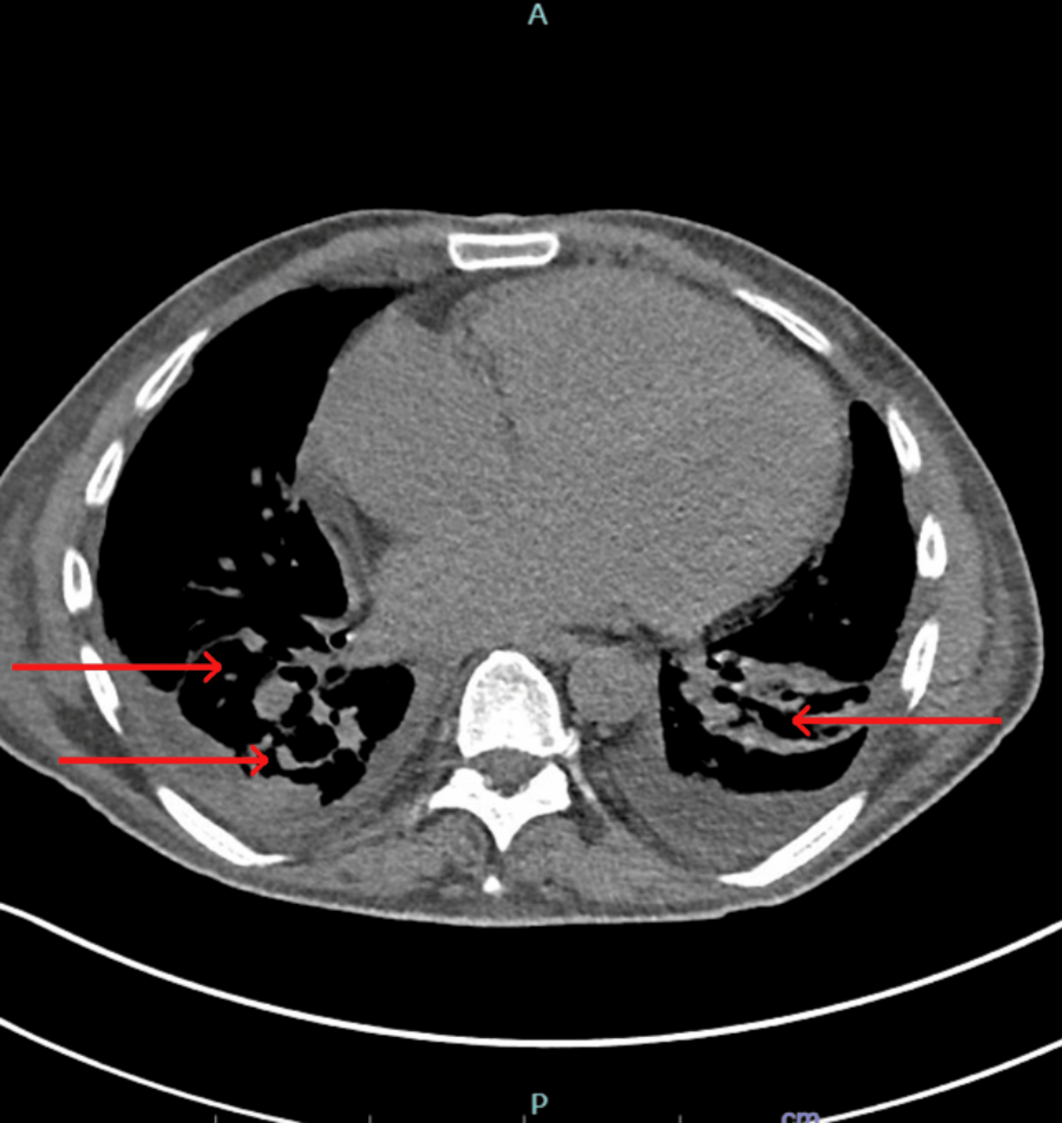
Non-contrast CT chest showing bilateral pleural effusions and patchy ground-glass infiltrates
